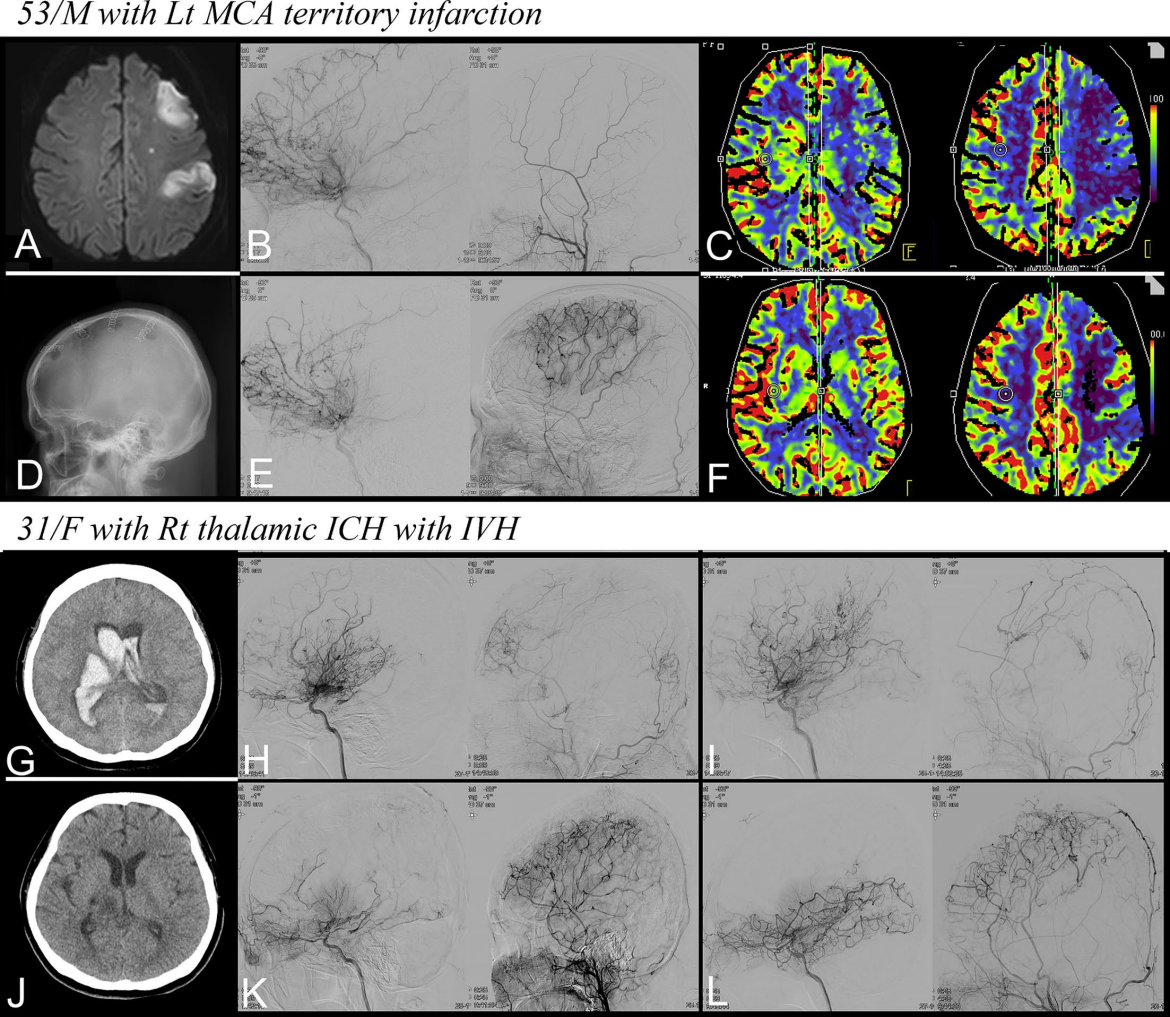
Representative cases. (A–F) A 53-year-old man with recent infarction in the left MCA territory. (A) Diffusion restriction in the left MCA territory, (B) Preoperative DSA for left ICA and ECA demonstrating moyamoya vessels, (C) Reduction in CBF on CTP, (D) Four multiple burr holes at the left hemisphere, (E) Follow-up DSA showing successful revascularization, (F) improvement in CBF on follow-up CTP. (G–L) A 31-year-old woman with right thalamic ICH with IVH. (G) Non-contrast CT at admission, (H,I) preoperative DSA for each hemisphere, (J) Non-contrast CT at discharge, and (K,L) Follow-up DSA showing successful revascularization. MCA, middle cerebral artery; ICA, internal carotid artery; ECA, external carotid artery; DSA, digital subtraction angiography; CBF, cerebral blood flow; CTP, CT perfusion; ICH, intracerebral hemorrhage; IVH, intraventricular hemorrhage; CT, computed tomography.
